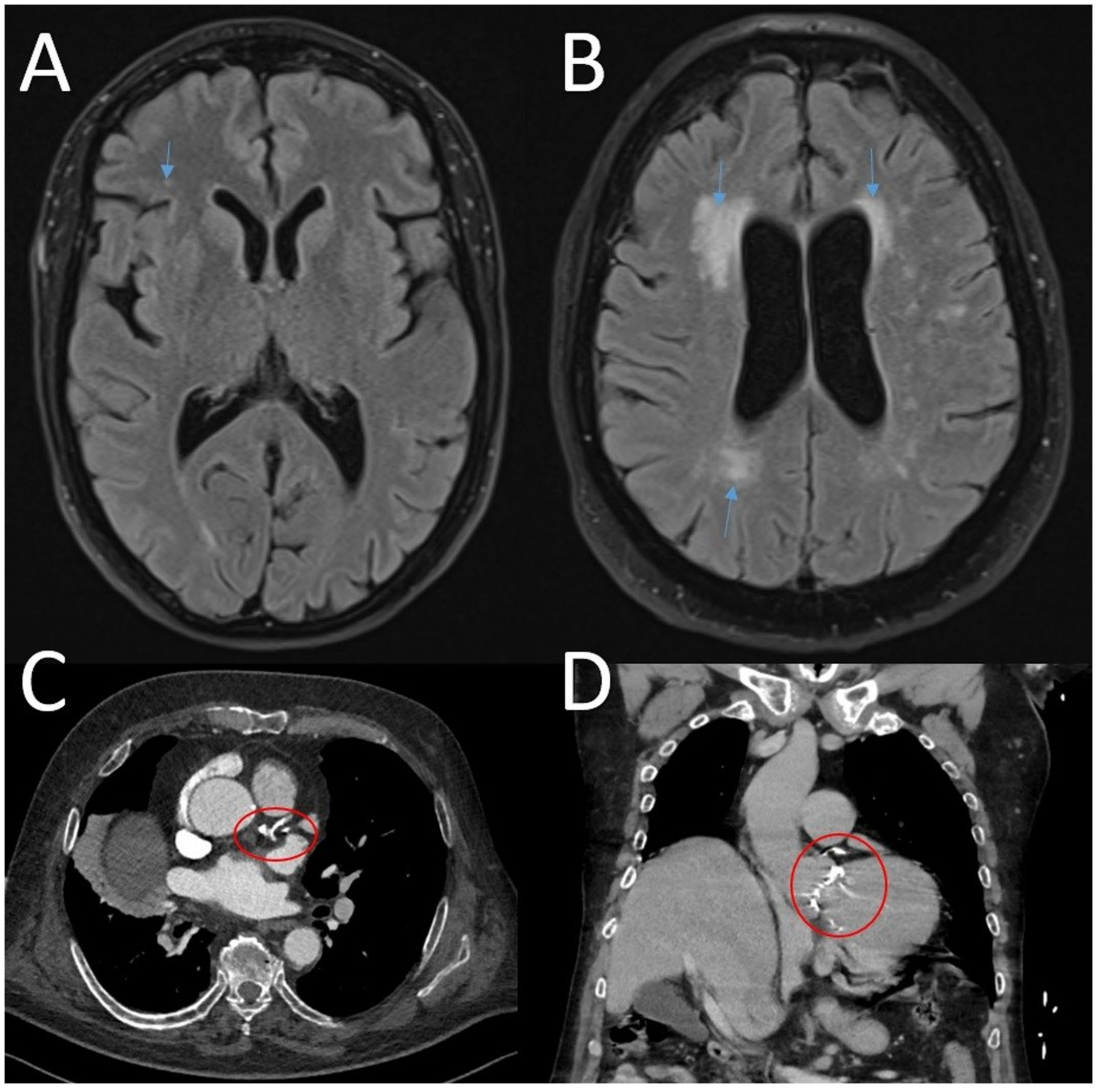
(A,B) MRI axial T2/FLAIR images of two patients. (A) Arrow points at single microvascular lesions (Fazekas score 1). (B) Arrows point at multiple confluent microvascular lesions (Fazekas score 2–3). (C,D) Contrast enhanced computed tomography scan showing severe coronary calcifications. (C) Axial. (D) Coronary.
